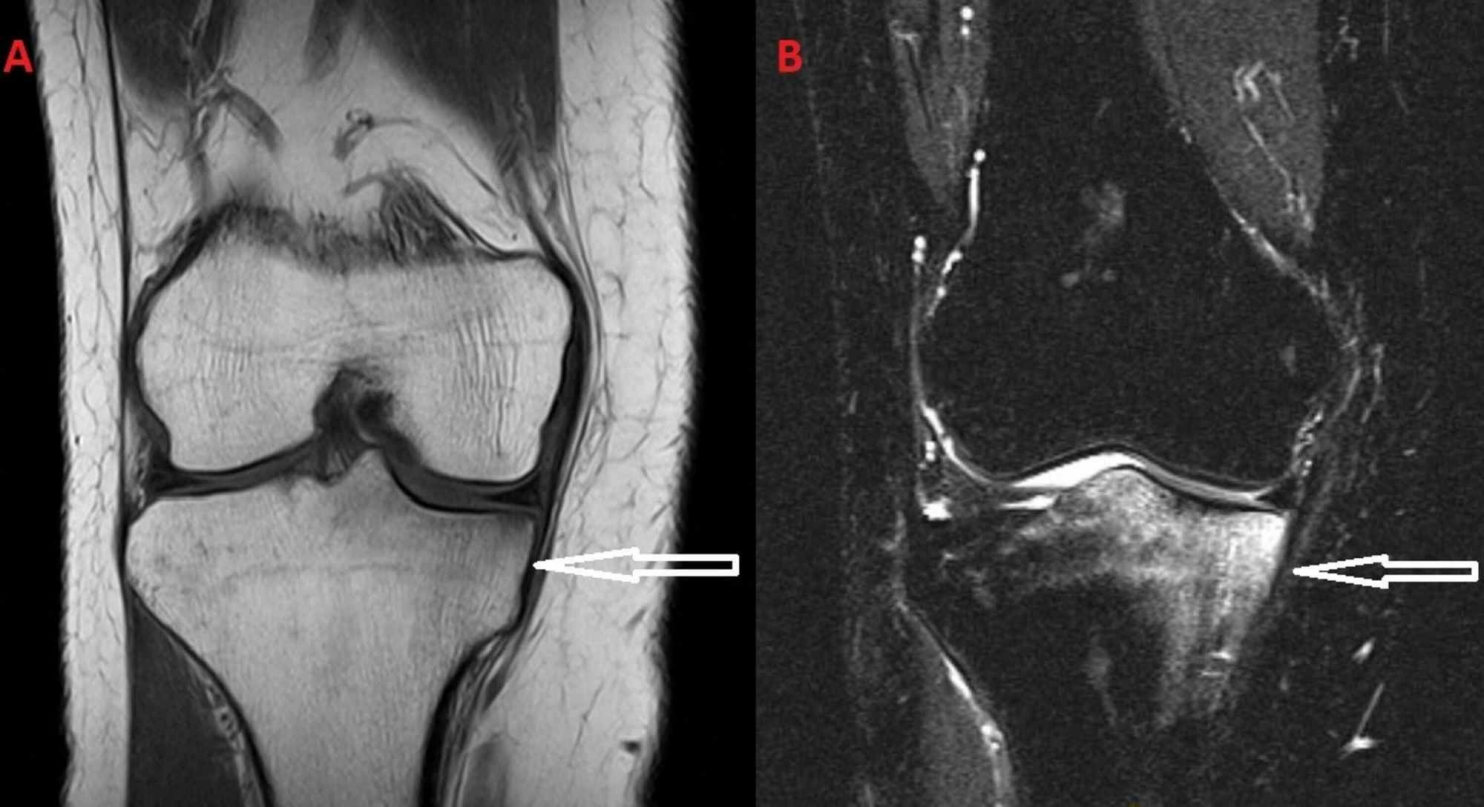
(A) T1-weighted images and (B) T2-weighted images from the initial MRI of the patient. The bone marrow edema is located at the medial tibial plateau.
